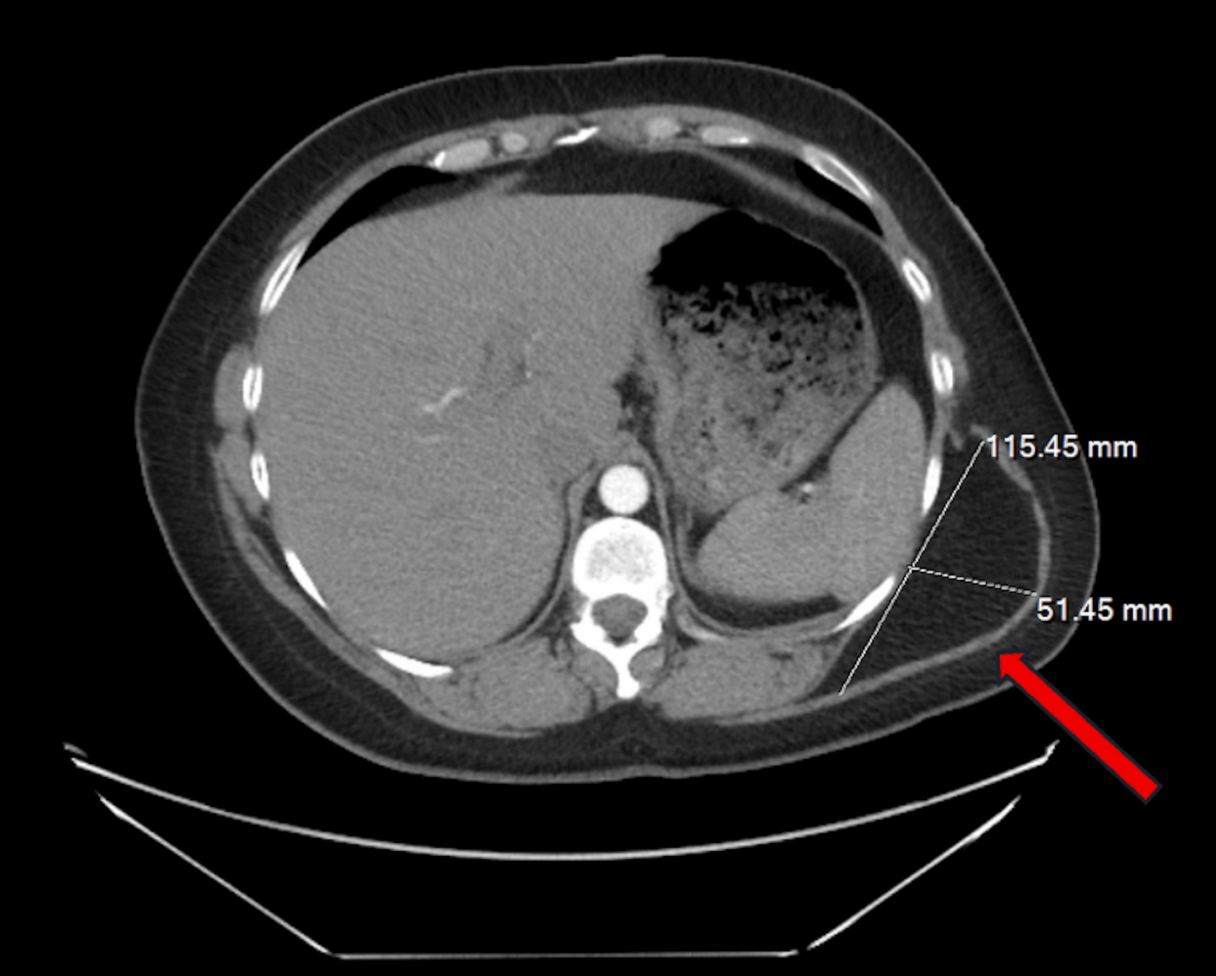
CT image of an incidental finding of a large lipoma measuring 11.5cm by 5.1cm, along the left posterior lateral flank region, deep to the latissimus dorsi muscle (arrow).
